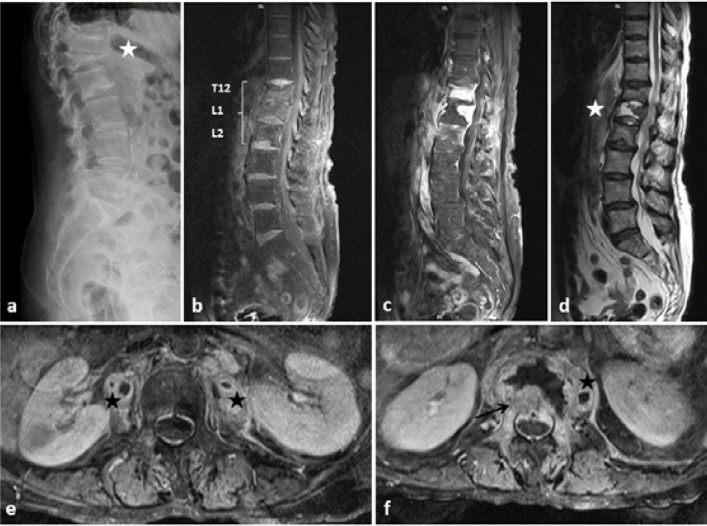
Imaging of the patient’s lumbar spine. (A) X-Ray of the lumbar spine showing compression of T12 and wedge-shaped deformity of L1 (white asterisk). (B-D) sagittal MRI lumbar spine. (B) T1-weighted MRI image of the lumbar spine and (C) fat-saturated T1-weighted MRI image (STIR) with post-contrast enhancement of the signal in the affected vertebrae and the surrounding tissues. (D) T2- weighted image (fluid signal - asterisk) differentiating the finding from metastatic lesions. (E-F) Axial MRI lumbar spine, black asterisks point to psoas abscesses. (F) Black arrow points to destruction of the vertebral body.
